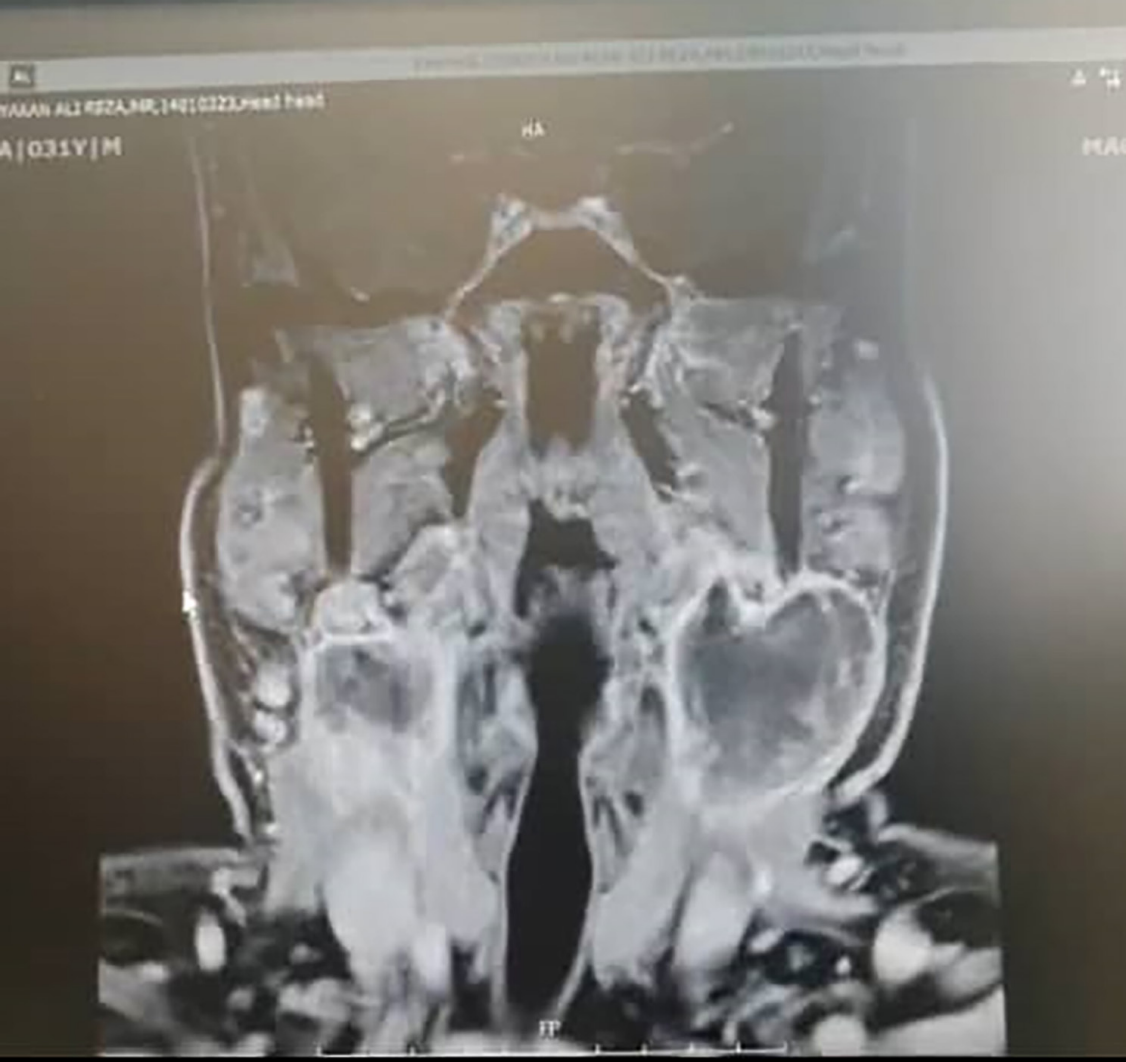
MRI view of parotid and submandibular glands.
